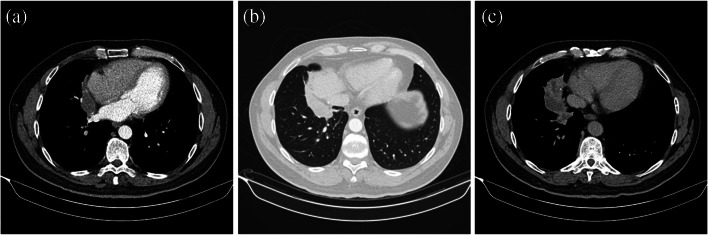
Computed tomography images of an intralobar PS adhered to the (a) pericardium and (b) diaphragm. c The computed tomography shows the mass to be of varying densities
